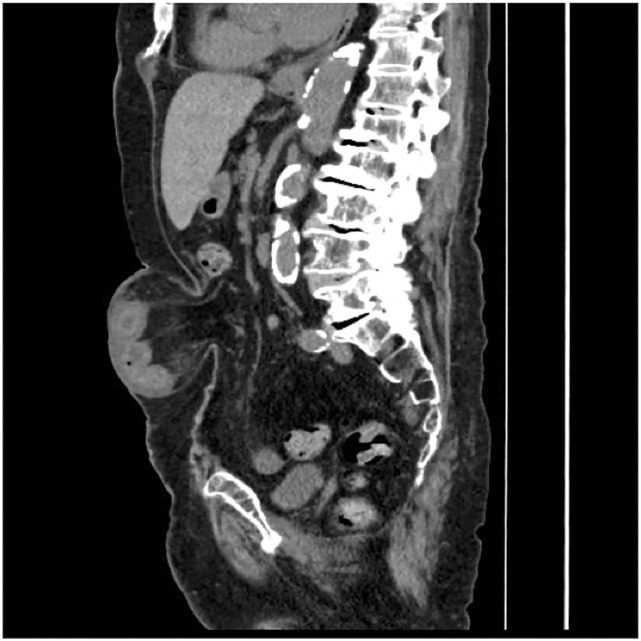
Preoperative CT (coronal view) of the abdomen. A 38 × 36-mm umbilical hernia with an acutely irreducible small bowel loop was observed.
